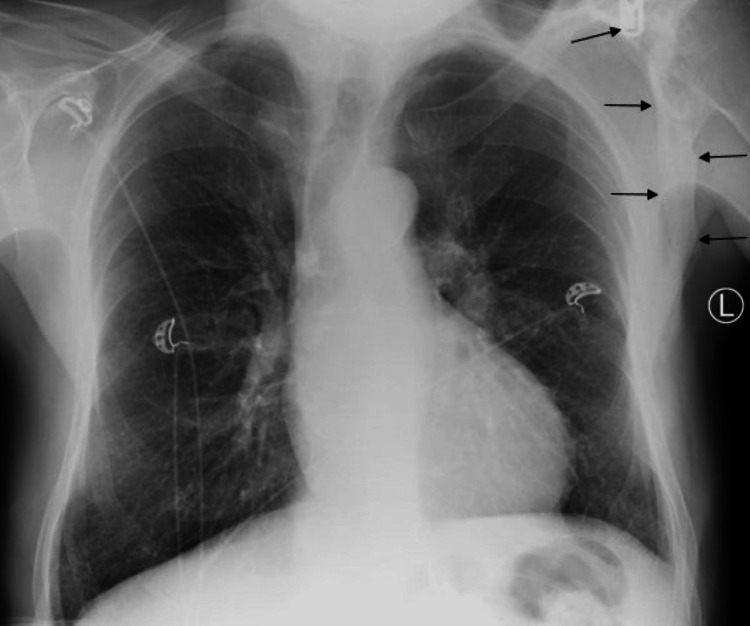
Chest radiograph PA view demonstrating bony enlargement of the left scapula with trabecular thickening (black arrows) PA, posterior anterior
